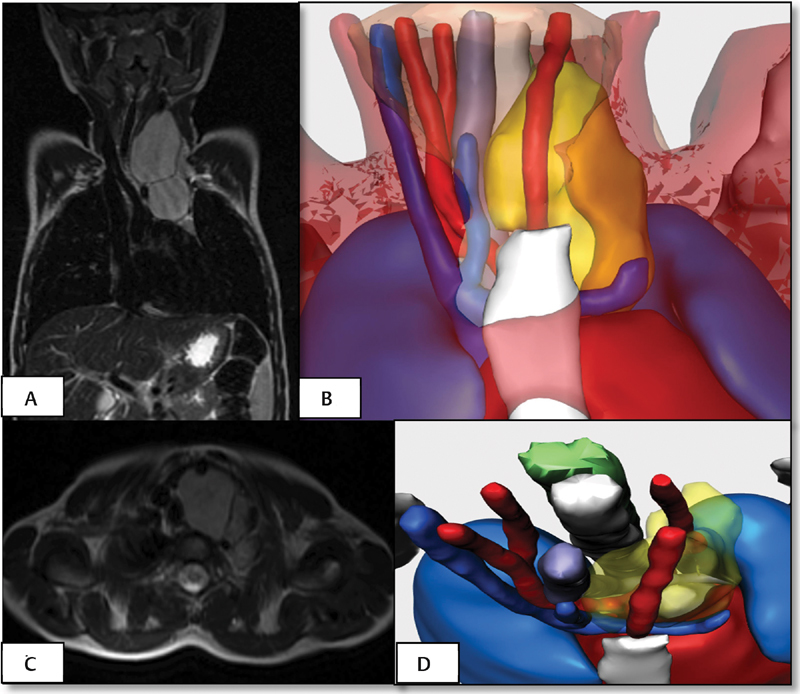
Case 4. Virtual reconstruction of cervicothoracic paravertebral ganglioneuroma and comparison with images obtained by MRI (A-B coronal view, C-D transverse view). The tumor displaces the left carotid artery, the internal jugular vein, and the airway. The vertebral artery is encased by the tumor. MRI, magnetic resonance imaging.
